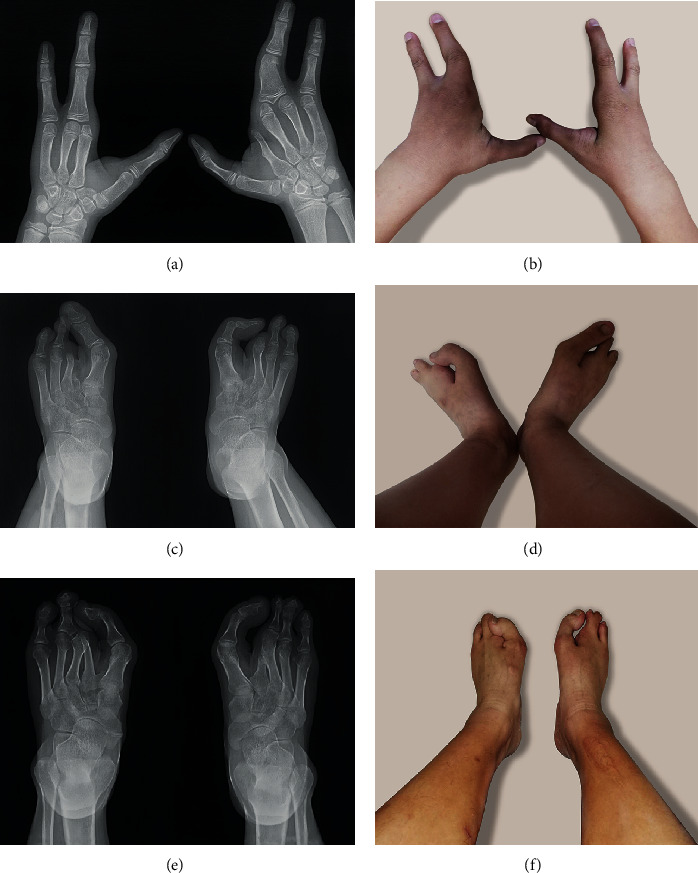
(a–d) Clinical phenotypes and X-rays of the proband's son (III-1). (e, f) Clinical phenotypes and X-rays of the proband (II-2).
